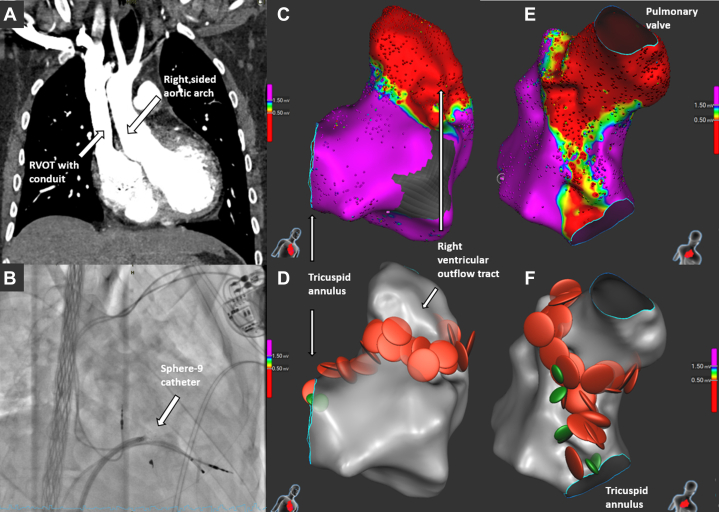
Findings on CT Angiography and Ablation Procedure in Case 4 (A) CT angiography anterior view showing right-sided aortic arch and RVOT conduit in a patient with double-outlet right ventricle and tetralogy physiology. (B) Right anterior oblique view showing the Sphere-9 catheter in the right ventricle. (C to F) RVOT scar (lateral and septal views) and RFA (red dots) and PFA (green dots) lesions encircling the scar boundary and connecting it to the tricuspid annulus. CT = computed tomography; PFA = pulsed-field ablation; RFA = radiofrequency ablation; RVOT = right ventricular outflow tract.
